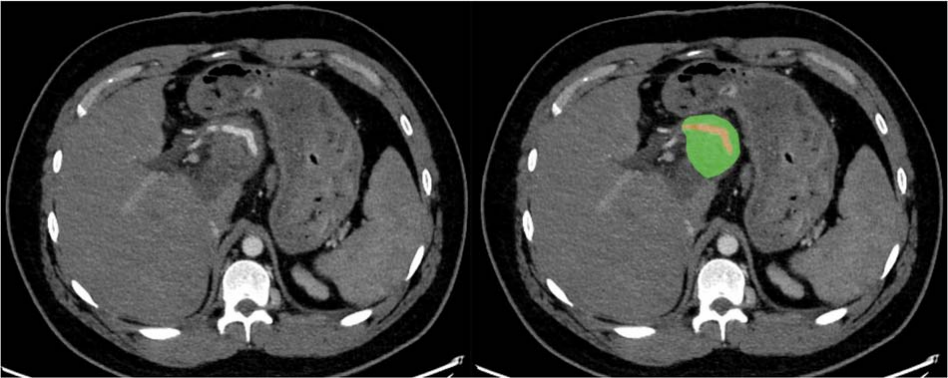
Axial section of CT with arterial contrast showing the hepatic artery (red) traversing within the superior aspect of the lesion (green).
